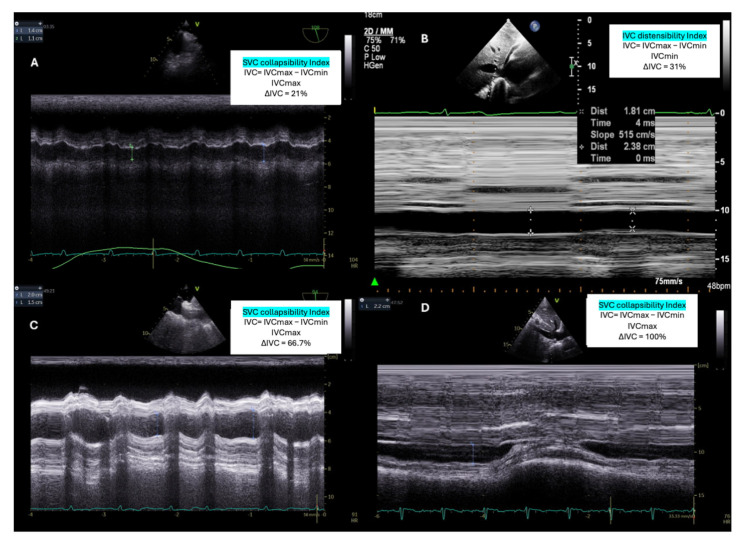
(A,C): Superior vena cava collapsibility index measured with transesophageal echocardiography. The reported threshold to identify fluid responsiveness varies in the literature between 18 and 36%. Patient (A) is probably not a fluid responder. On the contrary, patient (C) will respond to fluid administration. (B): IVC distensibility index in a fully sedated patient under controlled mechanical ventilation. The value of 31% indicates fluid responsiveness (D): IVC collapsibility index in a spontaneously breathing patient. The value of 100% indicates fluid responsiveness, although it should be considered with caution in a patient with rigorous respiratory efforts.
